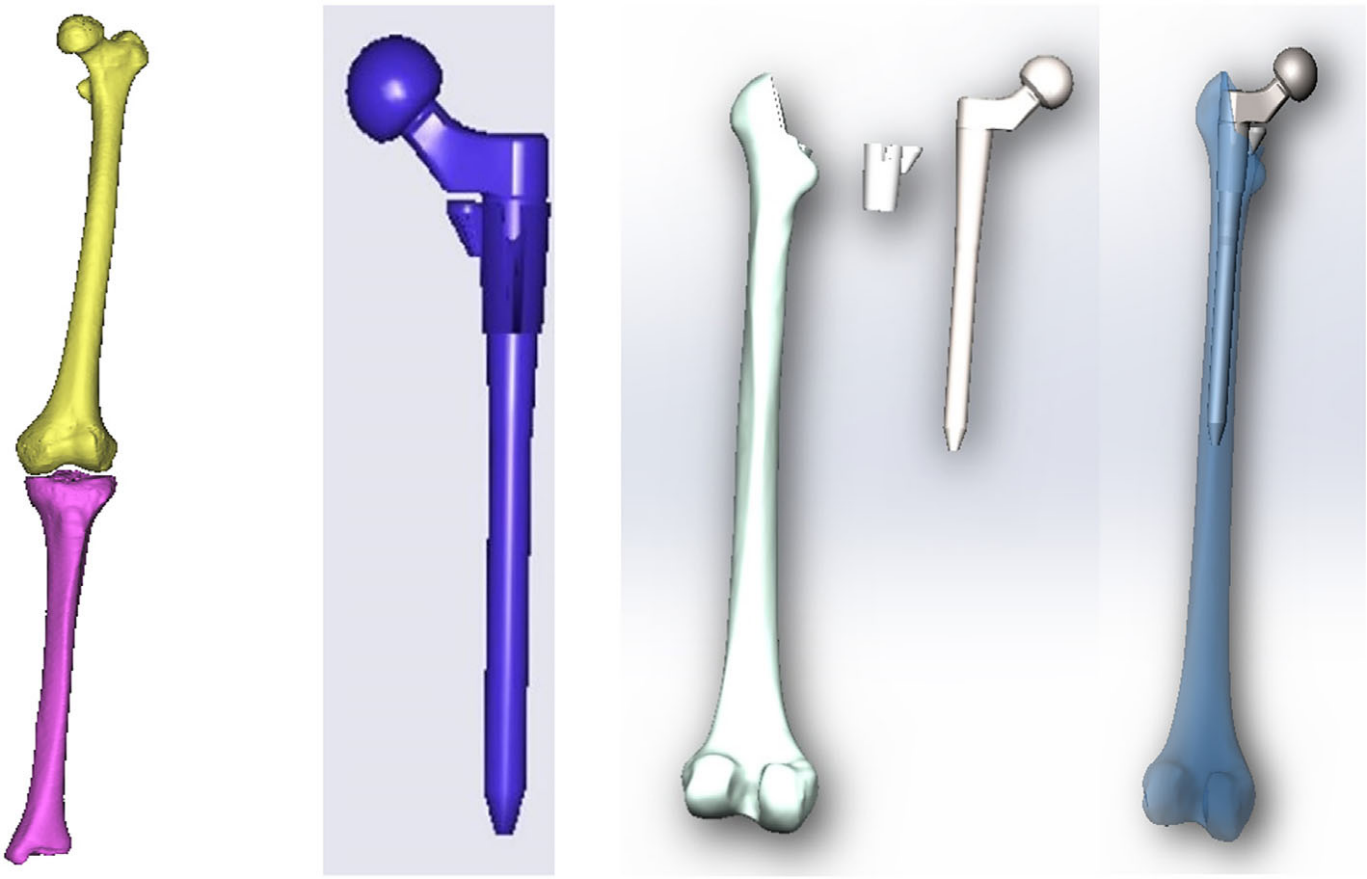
The CAD models of the femur and the S‐ROM hip implants were reestablished based on CT images of the female patient and realgeometry of the implanted BDS/F‐1/I hip implant, respectively: (A) left femur and tibia; (B) S‐ROM hip implant; (C) assembly model
